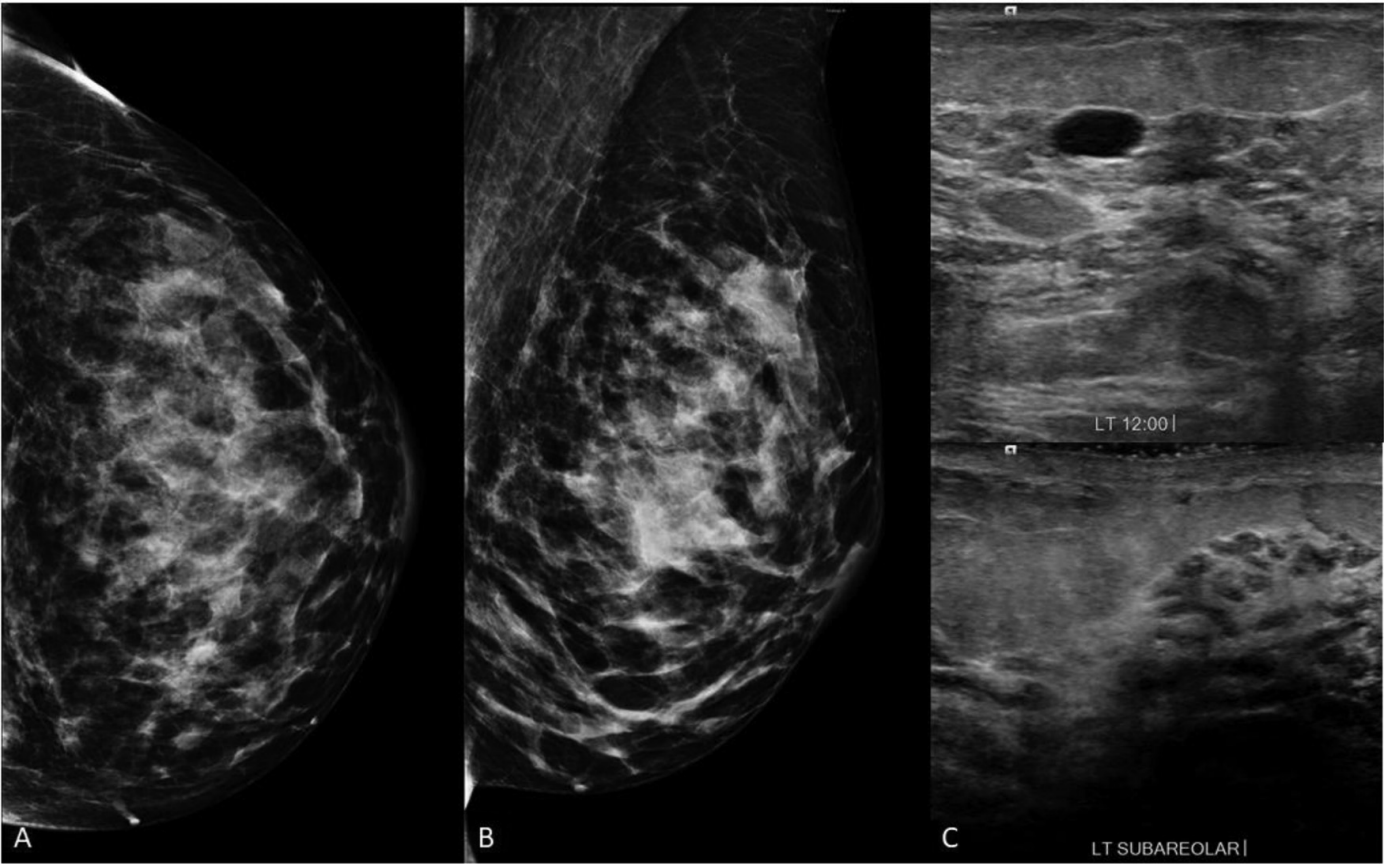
Initial diagnostic imaging in a 37-year-old female with spontaneous bloody left nipple discharge. (A) Craniocaudal (CC) and (B) mediolateral oblique (MLO) views demonstrate multiple bilateral oval circumscribed masses in both breasts (right breast not shown). No abnormality is seen in the subareolar region. (C) Representative image from the diagnostic ultrasound examination demonstrates one of the numerous cysts present in the left breast. No sonographic abnormality was seen in the subareolar left breast.
